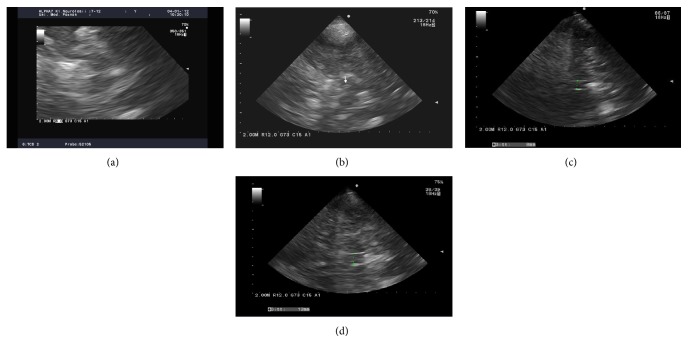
Ultrasound examination of the substantia nigra in a representative control (a) and patient with Parkinson's disease (PD) (b). The arrow in (b) indicates hyperechogenicity. The vertical green dotted line indicates normal 3rd ventricle in the healthy participant (c) and dilated 3rd ventricle in the patient with PD (d).
